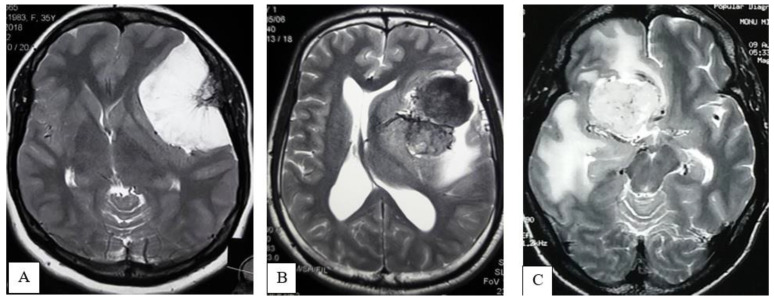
MRI of brain T2WI axial section demonstrates Absent peritumoral edema (A), Focal edema (B), Lobar or hemispheric edema (C).
